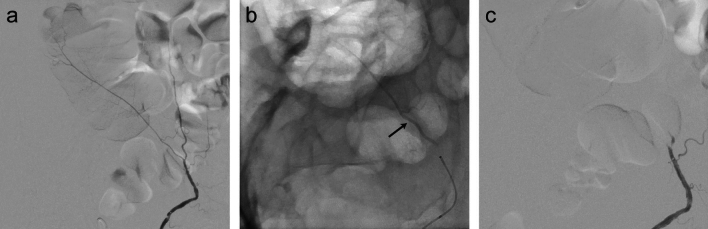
Embolization of the inferior epigastric artery for acute peripheral hemorrhage in a 57-year-old man with end-stage renal disease on hemodialysis, heart failure with reduced ejection fraction, polysubstance use, and newly diagnosed cirrhosis complicated by ascites underwent two bedside paracenteses, the second of which produced 3L of blood-tinged fluid accompanied by a drop in hemoglobin (6.0 g/dL from 7.8 g/dL). Vital signs were stable; however, computed tomography of the abdomen showed hemoperitoneum with a right rectus sheath hematoma and active arterial bleeding. (A) Pre-embolization anteroposterior digital subtraction angiography of the right inferior epigastric artery via a 4 French angled catheter via transfemoral access. (B) Fluoroscopic still image showing < 1 cc conformable embolic delivered via a 2.8 French microcatheter (arrow). (C) Post-embolization digital subtraction angiography demonstrating a static contrast column
